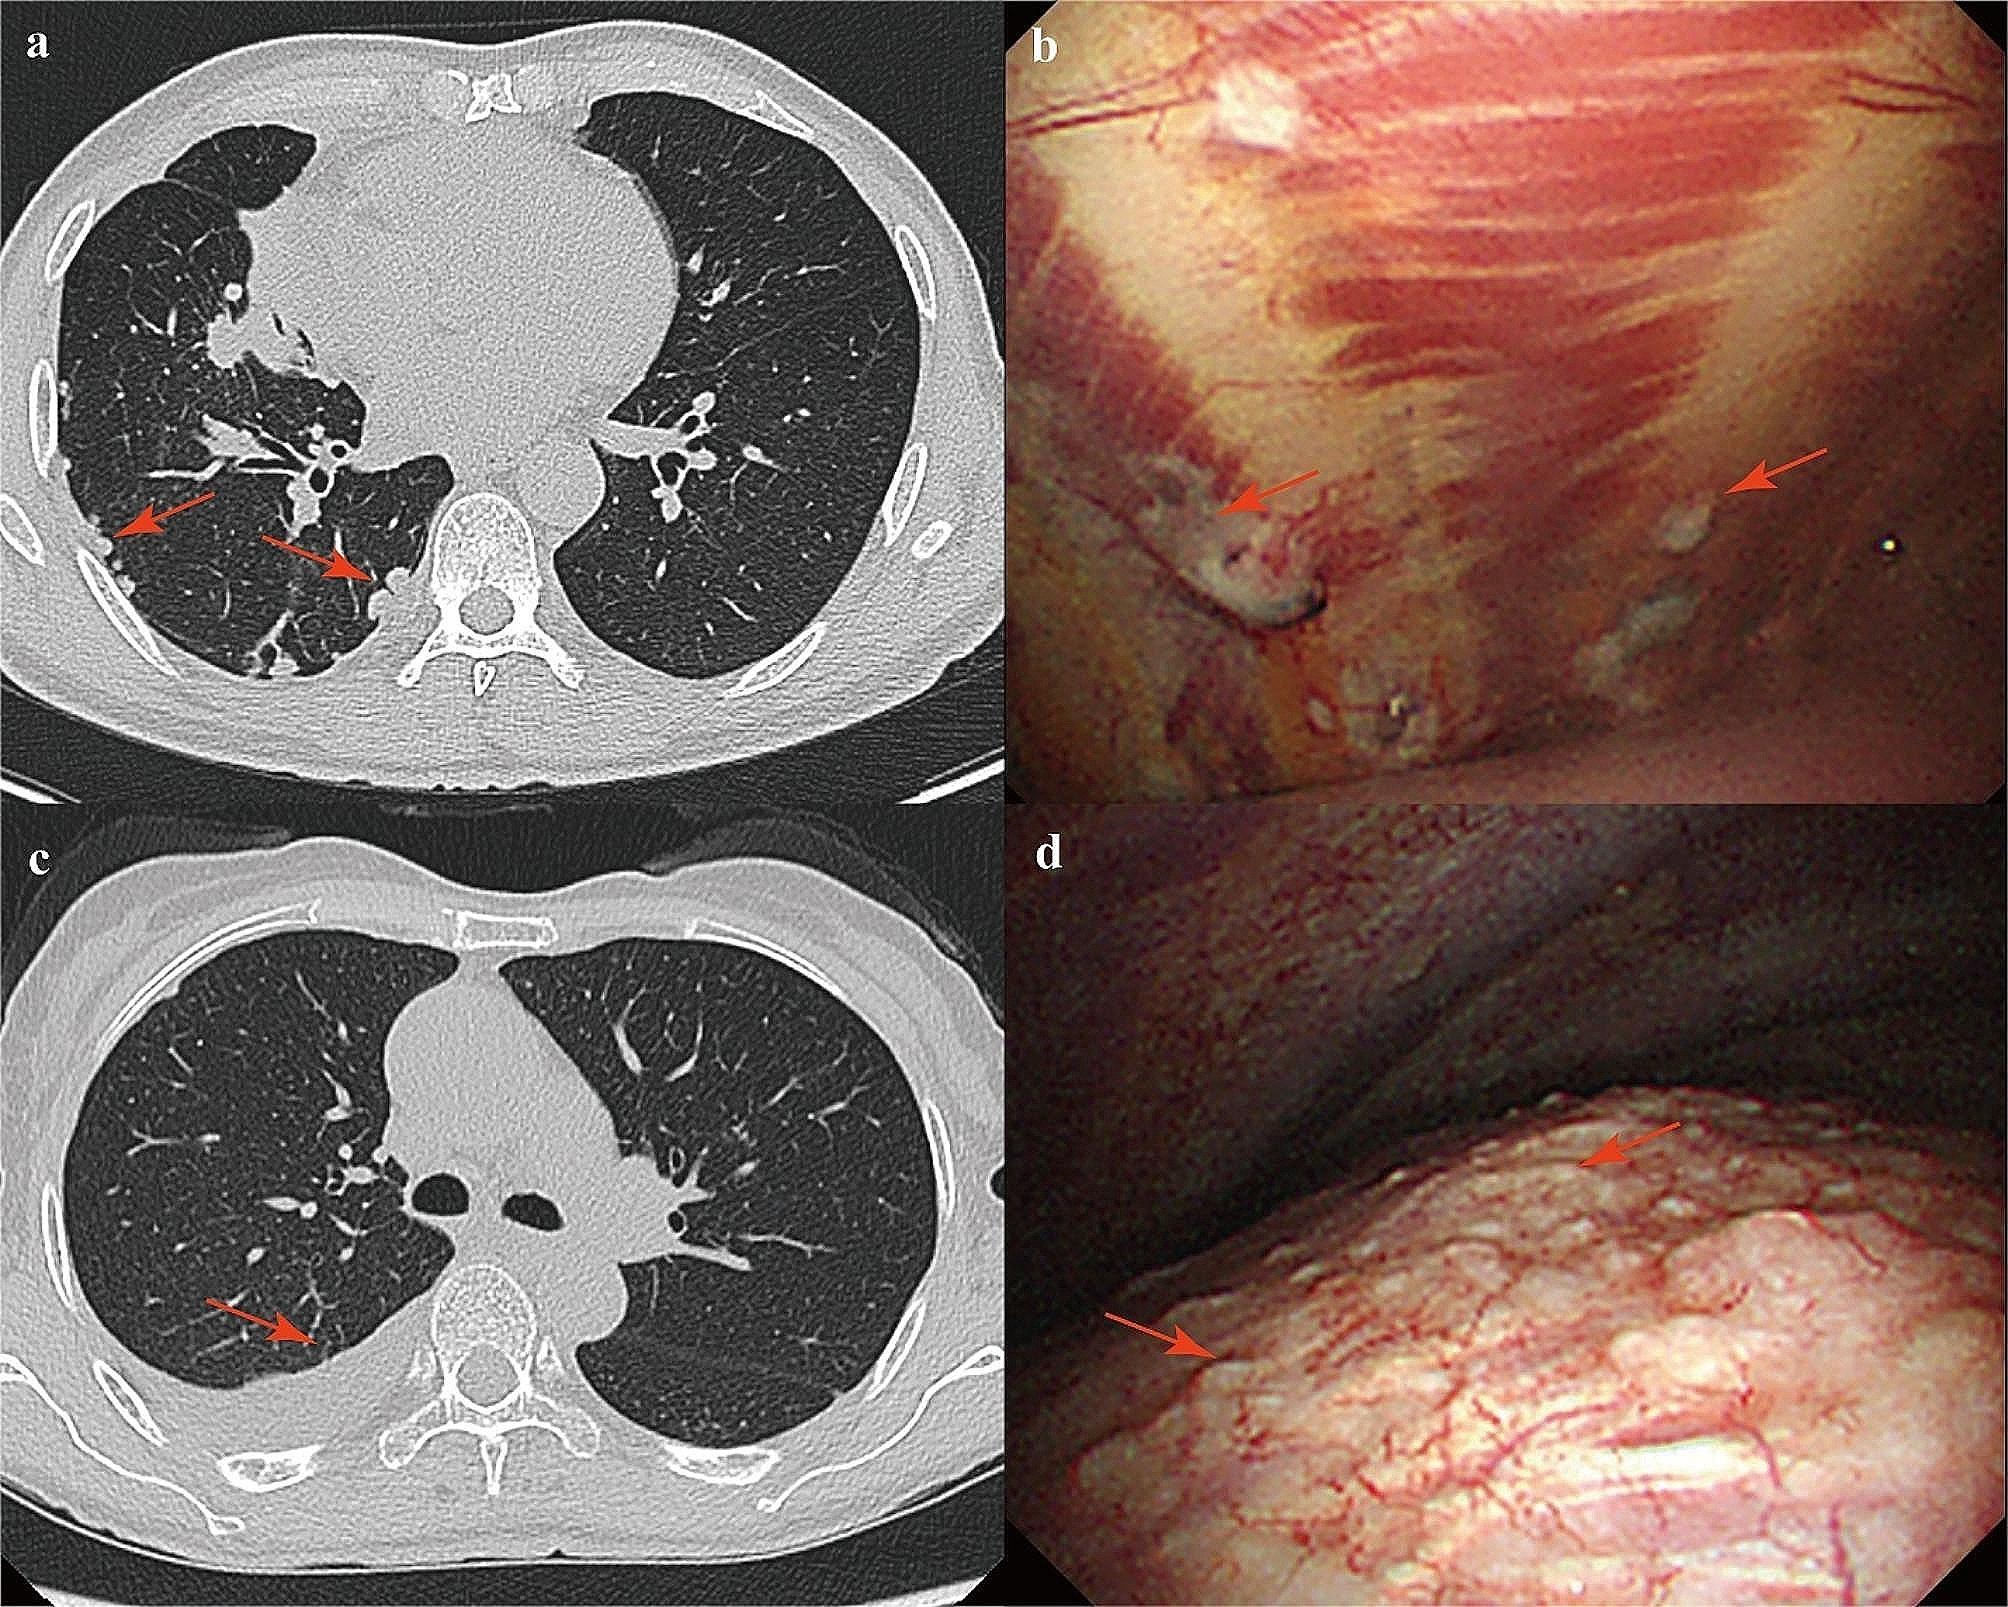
Thoracic CT scan and thoracoscopic presentation of typical patients. (a) Thoracic CT scan presentation in the patient with multiple nodules. (b) Thoracoscopic manifestation in the patient with multiple pleural nodules. He was diagnosed with pleural metastasis from lung cancer. (c) Thoracic CT scan of a patient with tuberculous pleurisy showed a small amount of effusion in the right pleural cavity. (d) Thoracoscopic manifestation in the patient showed multiple nodules in the diaphragm pleura, and he was diagnosed with tuberculous pleurisy
